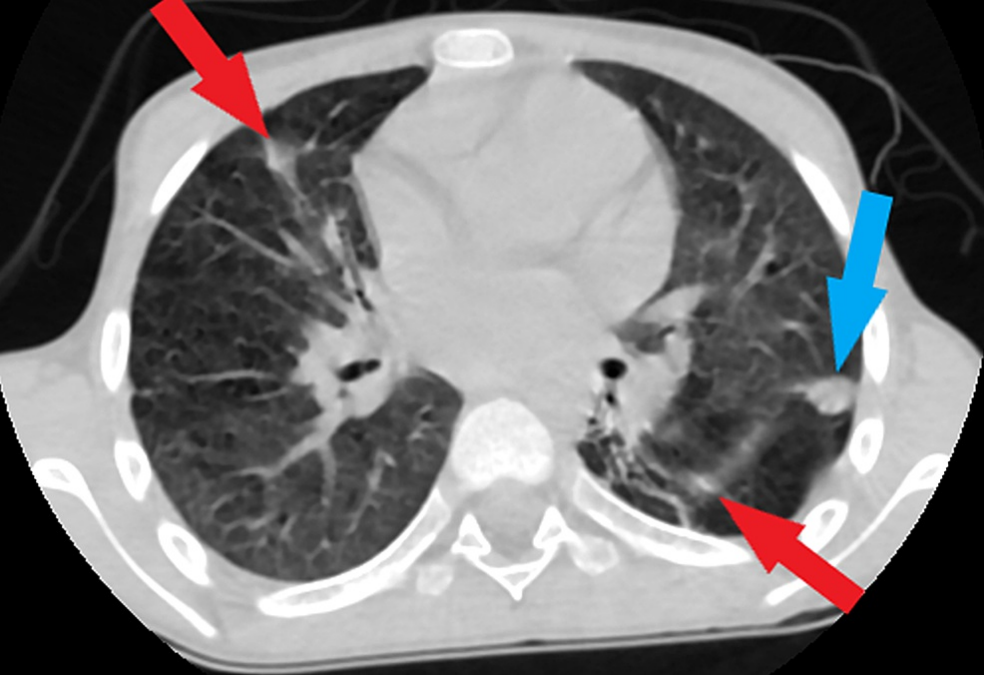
CT scan of the chest, axial view with no contrast showing multiple linear opacities in both lungs suggestive of bilateral linear atelectasis (red arrows) and a single solitary lung nodule in left lung measures 10*11 mm (blue arrow).
